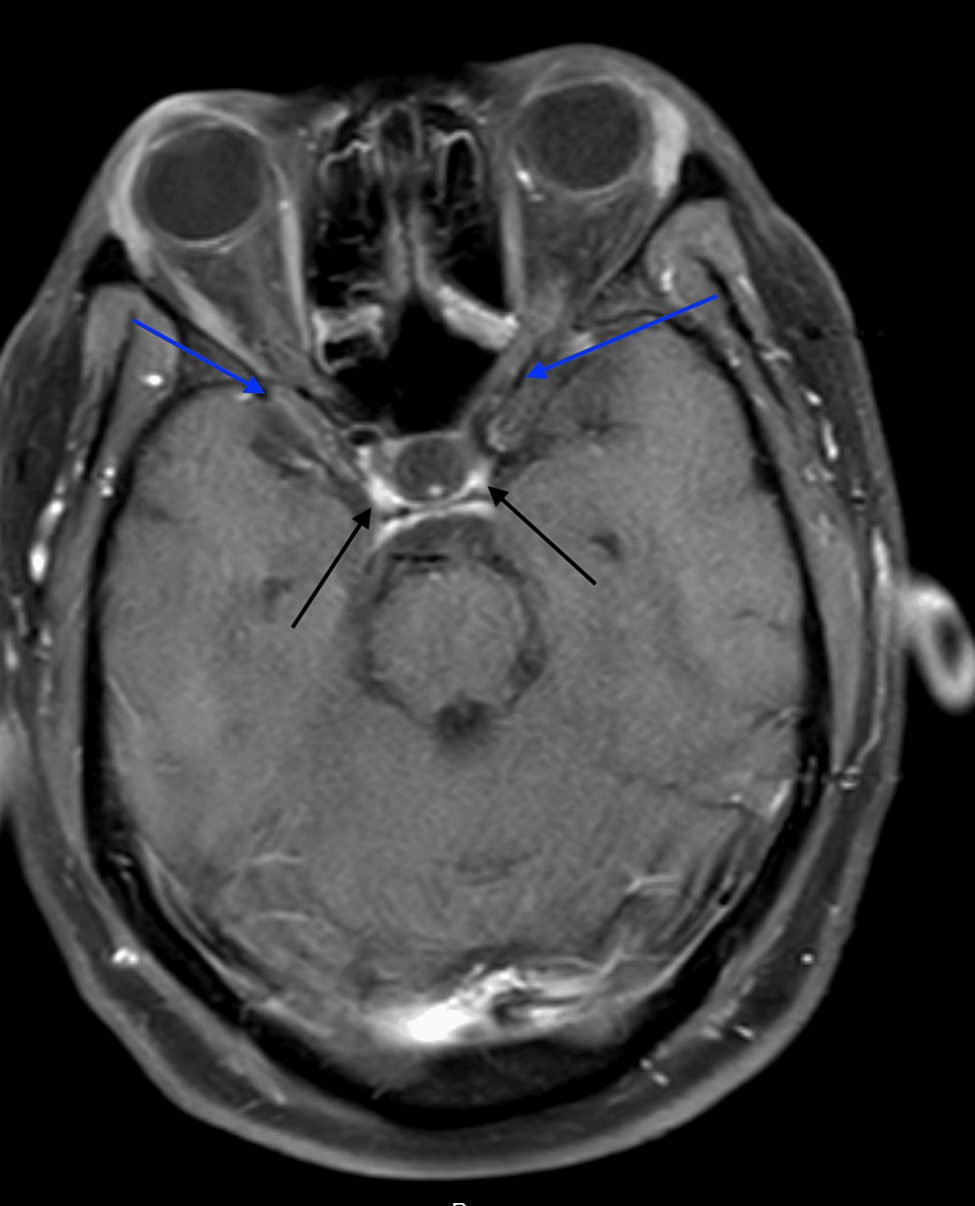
MRI of the brain with contrast showing abnormal enhancement of the posterior portions of the bilateral optic nerves (blue arrows) and optic chiasm (black arrows)
